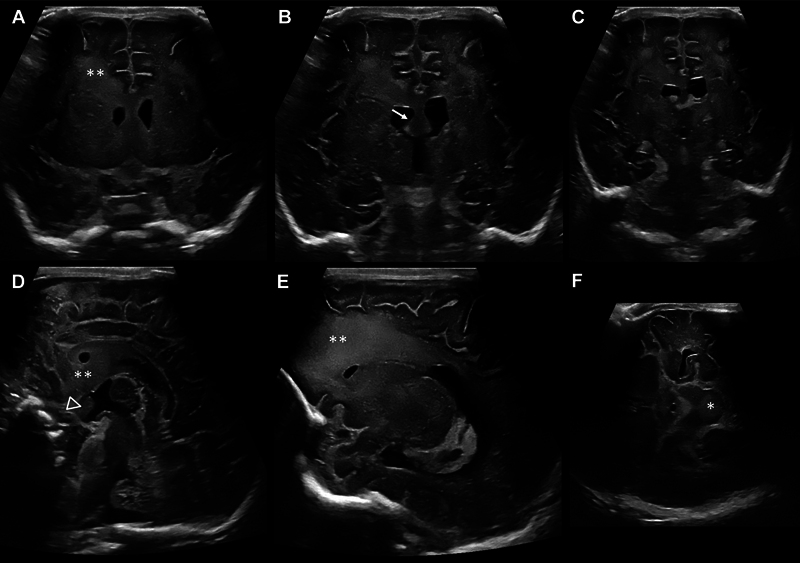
Cranial ultrasound on DOL 11. Increase of white matter echogenicity with very fine, homogeneous echo texture (two asterisks) with frontal predominance (
